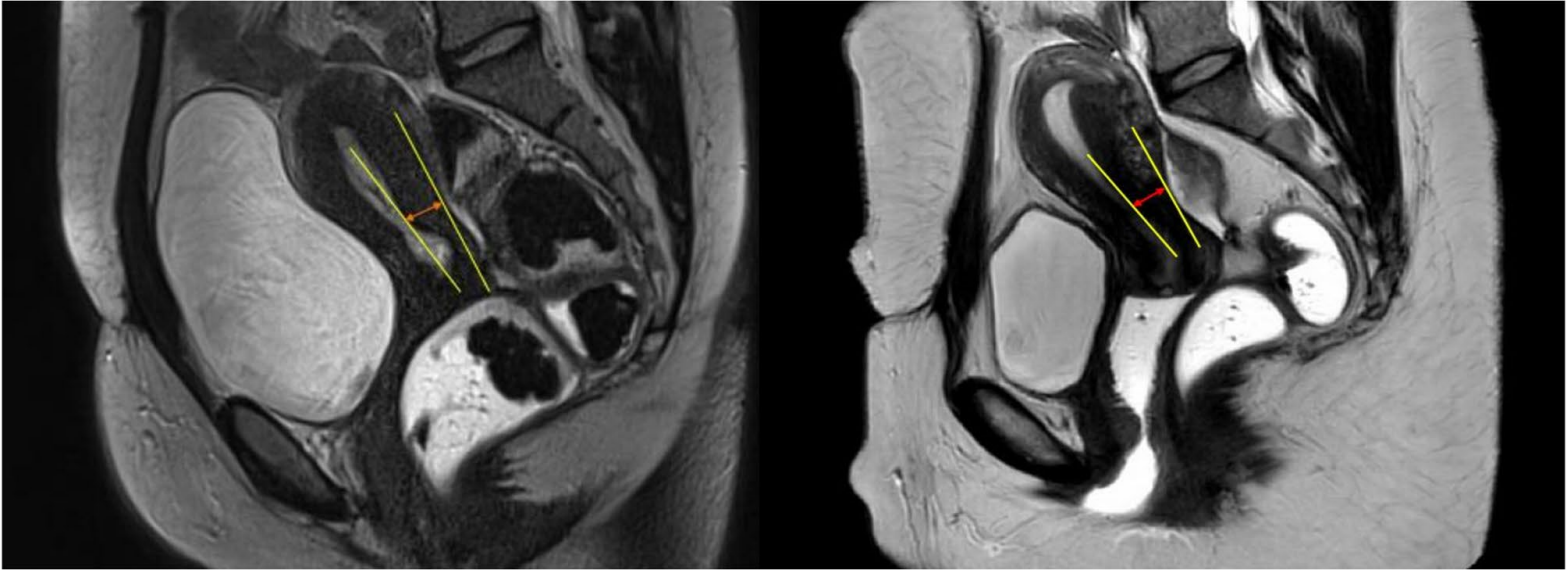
Examples of posterior isthmus thickness measurement on sagittal T2-weighted pelvic MRI. Yellow solid line: posterior uterine wall axis (endometrial and peritoneal edge) / Red arrow: posterior uterine isthmus thickness (millimeter)
